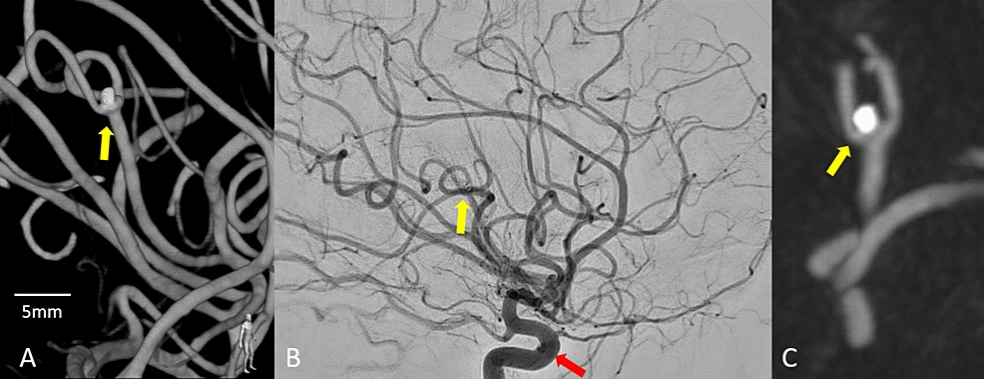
12-day post-procedural cerebral angiogram 12-day post-procedural cerebral angiogram confirming complete coil embolization of the ruptured left MCA posterior trunk M3 bifurcation aneurysm (yellow arrow), with unremarkable patency of the parent arteries. The round left ICA transitional aneurysm (red arrow), again unchanged in size and morphology, compared with the previous three Cerebral Angiograms, the most remote in 2011. No new aneurysms were identified. (A 3D images; B angiographic images; C Vaso-CT images).
